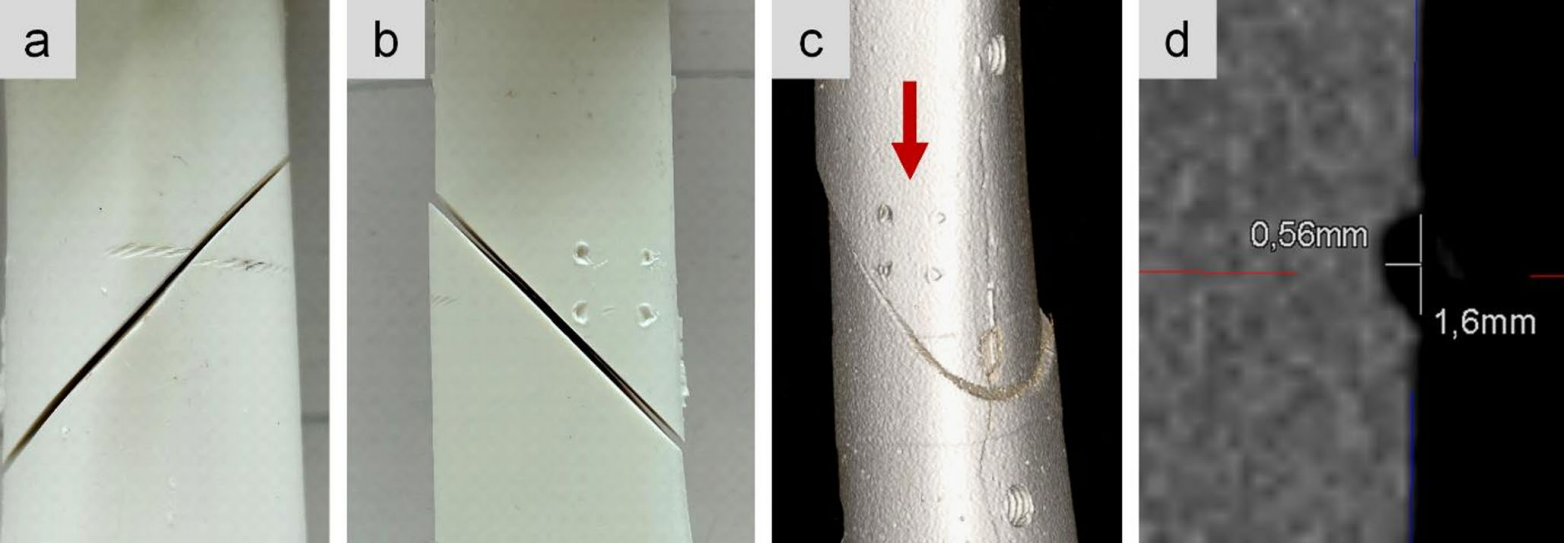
Visual inspection of cerclage imprints (a) and notches from the cerclage clamping (b) in a synthetic sample. In CT scans only the notches from the cerclage clamping were visible (c). Exemplary measurement of the notch size in synthetic bone (d)
